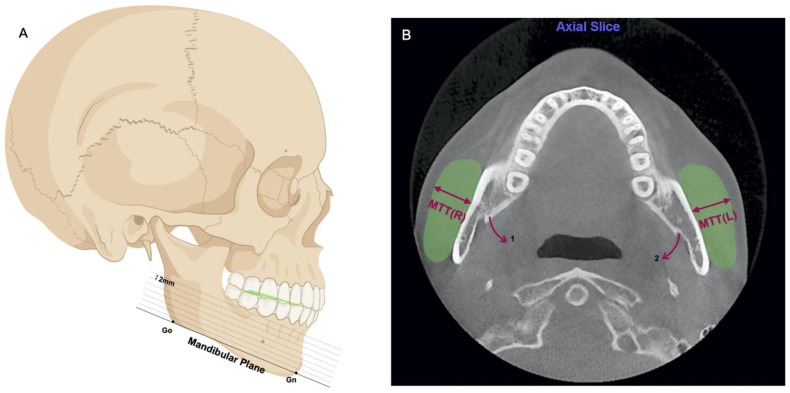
Measurement of the masseter muscle thickness (MTT). (A) The head position in the 3D reconstruction was required to be repositioned, ensuring the CBCT image was divided parallel to the mandibular plane (MP). The distance between each slice was 2mm; (B) The MMT of both sides was measured on the axial slice passing through the mandibular lingual structure (arrow 1), which is a sharp and thin bony piece in front of the mandibular foramen (arrow 2).
